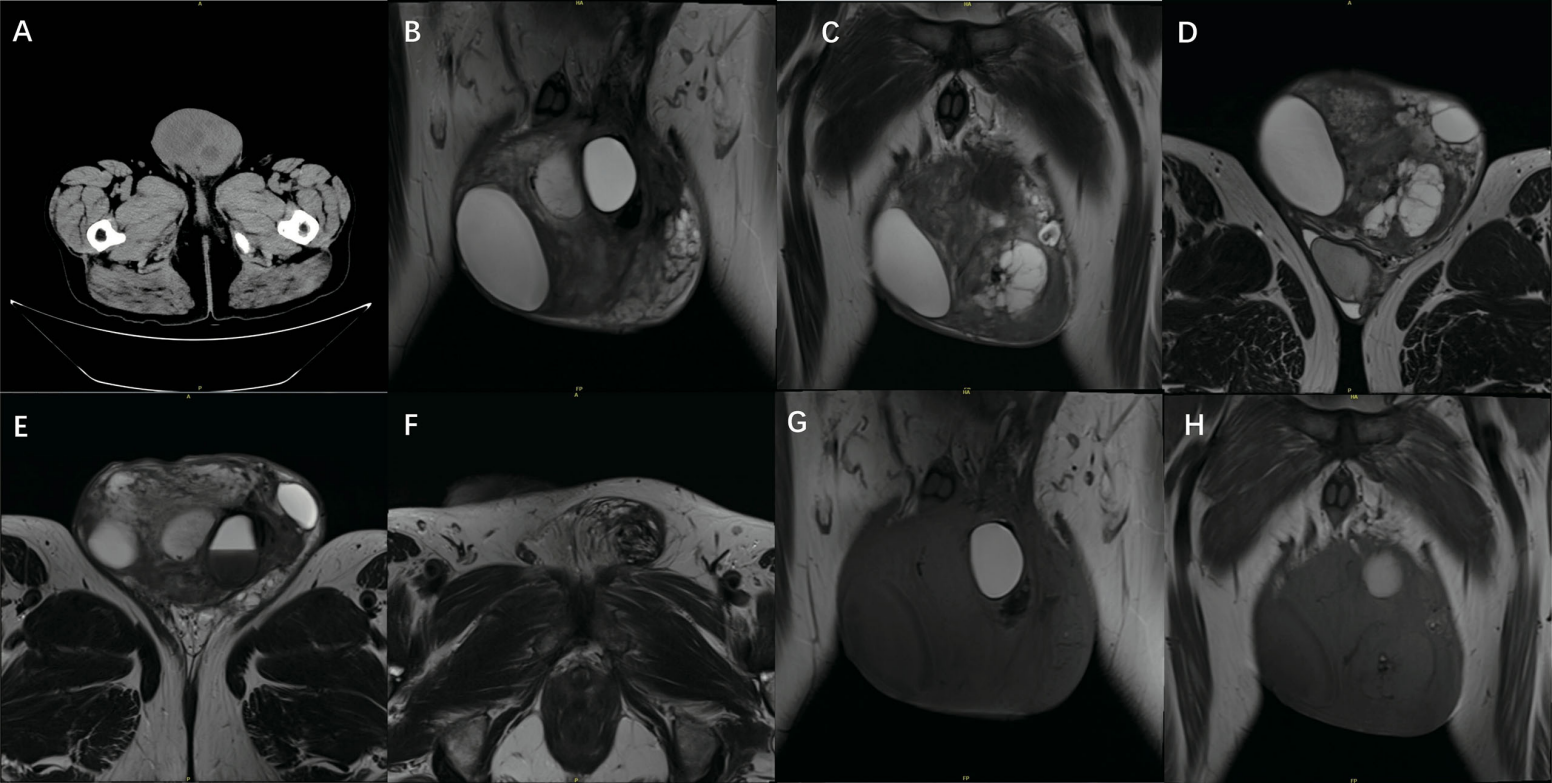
CT: (A): CT shows heterogeneous isometric, low-mixed tissue mass foci in the scrotum. MRI: (B): The left testicle is visible in the T2 coronal position. (C): T2 coronal view shows tumor tissue (D): T2 axial view shows tumor parenchyma, and the testis squeezed to the bottom. (E): T2 axial view shows the testis. (F): T2 axial view shows the mass extending to the inguinal region. (G, H) T1 coronal view shows tumor NMR signal.
